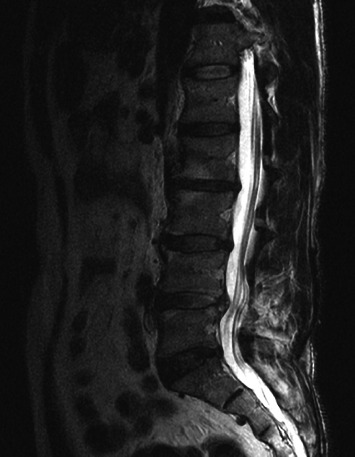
Lumbar spine magnetic resonance image shows unremarkable changes compared with the prediscography image findings.
